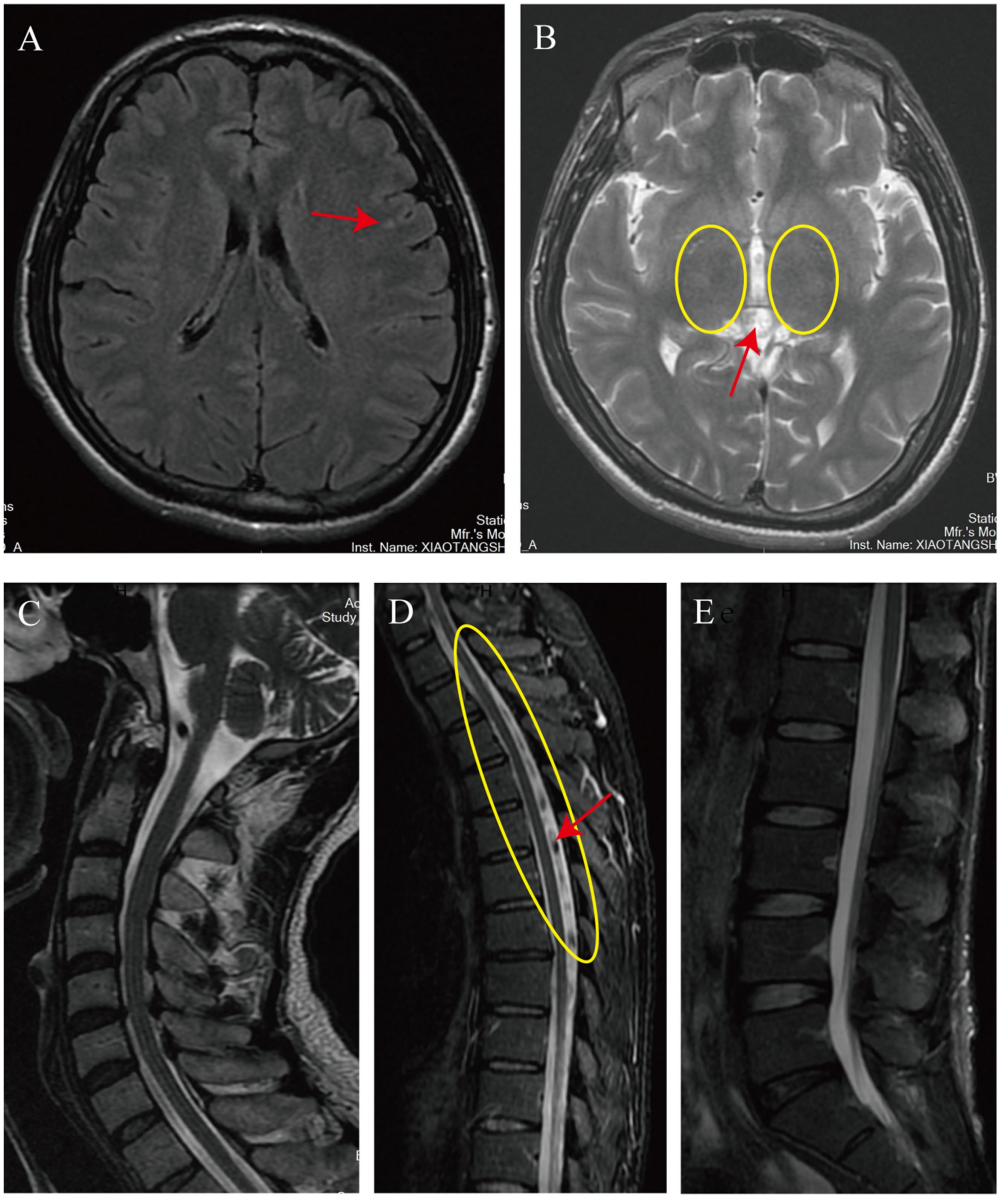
The imaging findings upon the patient’s admission. The cranial MRI reveals patchy T2-FLAIR hyperintense signals in the left frontal lobe (indicated by the red arrow in A). Additionally, it shows faintly hyperintense, slightly increased signals in the bilateral basal ganglia on T2-weighted images (encircled in yellow in B) and an isointense pineal gland with a diameter of approximately 11 mm (also indicated by the red arrow in A). The MRI of the entire cervical and lumbar spinal cord shows no significant abnormalities (C,E). However, the thoracic spine MRI demonstrates a tortuous and thickened capillary plexus within the dural sac on T2-weighted images from T2 to T8 (encircled in yellow in D), with some vessels exhibiting a poor flow void effect (indicated by the red arrow in D).
